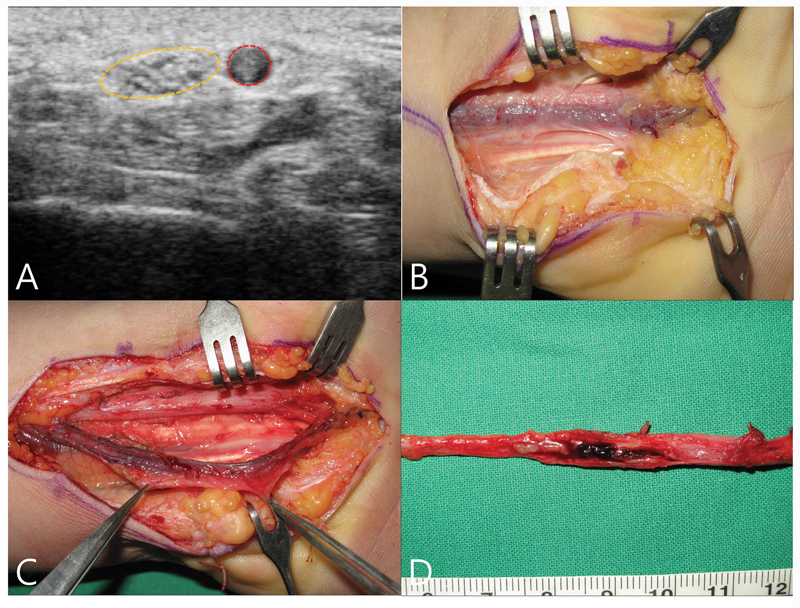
A 26-year-old man presented with a 1-week history of fingertip numbness and wrist pain, without trauma. Plain radiographs were unremarkable, while light-touch sensation was decreased in the median nerve territory. Tinel's sign and compression tests at the wrist were positive, but nerve conduction studies and electromyography were normal. (
